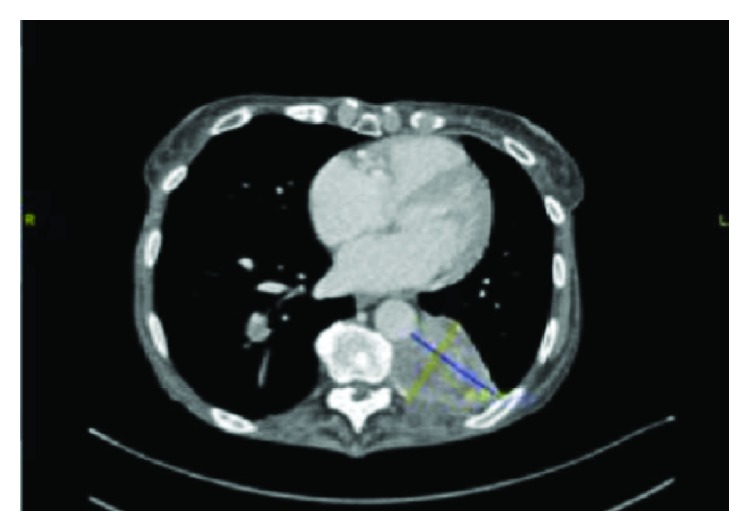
Restaging CT post 19 cycles of Nivolumab. There was a tumour regression in the RLL and LLL mass.
